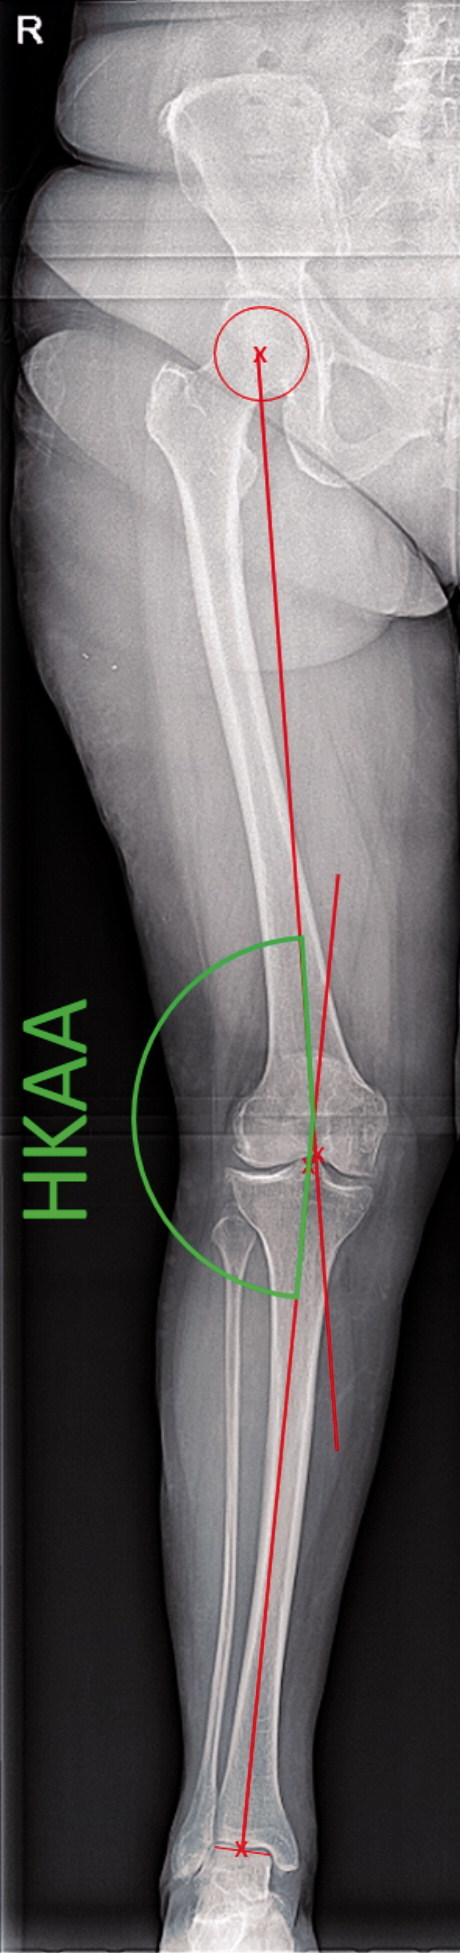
Measurement of the Hip–knee–ankle angle on full limb radiograph. The hip–knee–ankle angle (HKAA, in green) is measured between 2 axes (in red). One axis runs from the middle of the femoral head to the middle of the femoral notch, and a second axis from the middle of the tibial notch to the middle of the talar head. Definitions of the femoral axis from left to right (top): Fem1—mid-shaft at approximately 10 cm proximal of the femoral notch + mid-shaft in the area where the meta- and epiphysis meet (van Raaij et al. 2009, Iranpour-Boroujeni et al. 2014, Zampogna et al. 2015). Fem2—mid-shaft at approximately 10 cm proximal of the femoral notch + center of the femoral notch (Felson et al. 2009, van Raaij et al. 2009, McDaniel et al. 2010, Sheehy et al. 2011). Fem3—mid-shaft at approximately 10 cm proximal of the femoral notch + base of the tibial spines (Kraus et al. 2005, Hinman et al. 2006, Issa et al. 2007, McDaniel et al. 2010, Navali et al. 2012, Iranpour-Boroujeni et al. 2014, Zampogna et al. 2015). Fem4—mid-shaft at approximately 10 cm proximal of the femoral notch + middle of tibial plateau (McDaniel et al. 2010). Definitions of the tibial axis from left to right (bottom): Tib1—mid-shaft at approximately 10 cm distal of the base of the tibial spines + mid-shaft in the area where the meta- and epiphysis meet (van Raaij et al. 2009, Iranpour-Boroujeni et al. 2014, Zampogna et al. 2015). Tib2 Mid-shaft at approximately 10 cm distal of the base of the tibial spines + center of the femoral notch (McDaniel et al. 2010). Tib3—mid-shaft at approximately 10 cm distal of the base of the tibial spines + base of the tibial spines (Kraus et al. 2005, Hinman et al. 2006, Issa et al. 2007, Colebatch et al. 2009, van Raaij et al. 2009, McDaniel et al. 2010, Sheehy et al. 2011, Navali et al. 2012, Iranpour-Boroujeni et al. 2014, Zampogna et al. 2015). Tib4—mid-shaft at approximately 10 cm distal of the base of the tibial spines + middle of tibial plateau (McDaniel et al. 2010). The 2 pictures on the left show the measurement of the FTA using method 2 for the femoral axis and method 1 for the tibial axis on a standard AP knee radiograph from the present data set.
